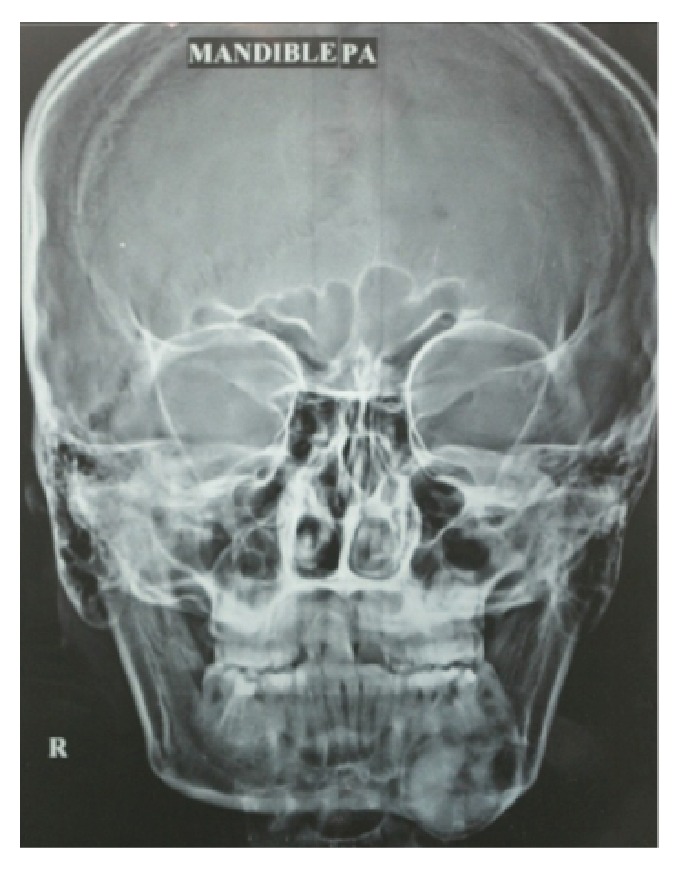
Posteroanterior radiograph showing homogenous radioopacity with thin radiolucent border in the left mandibular posterior region.
